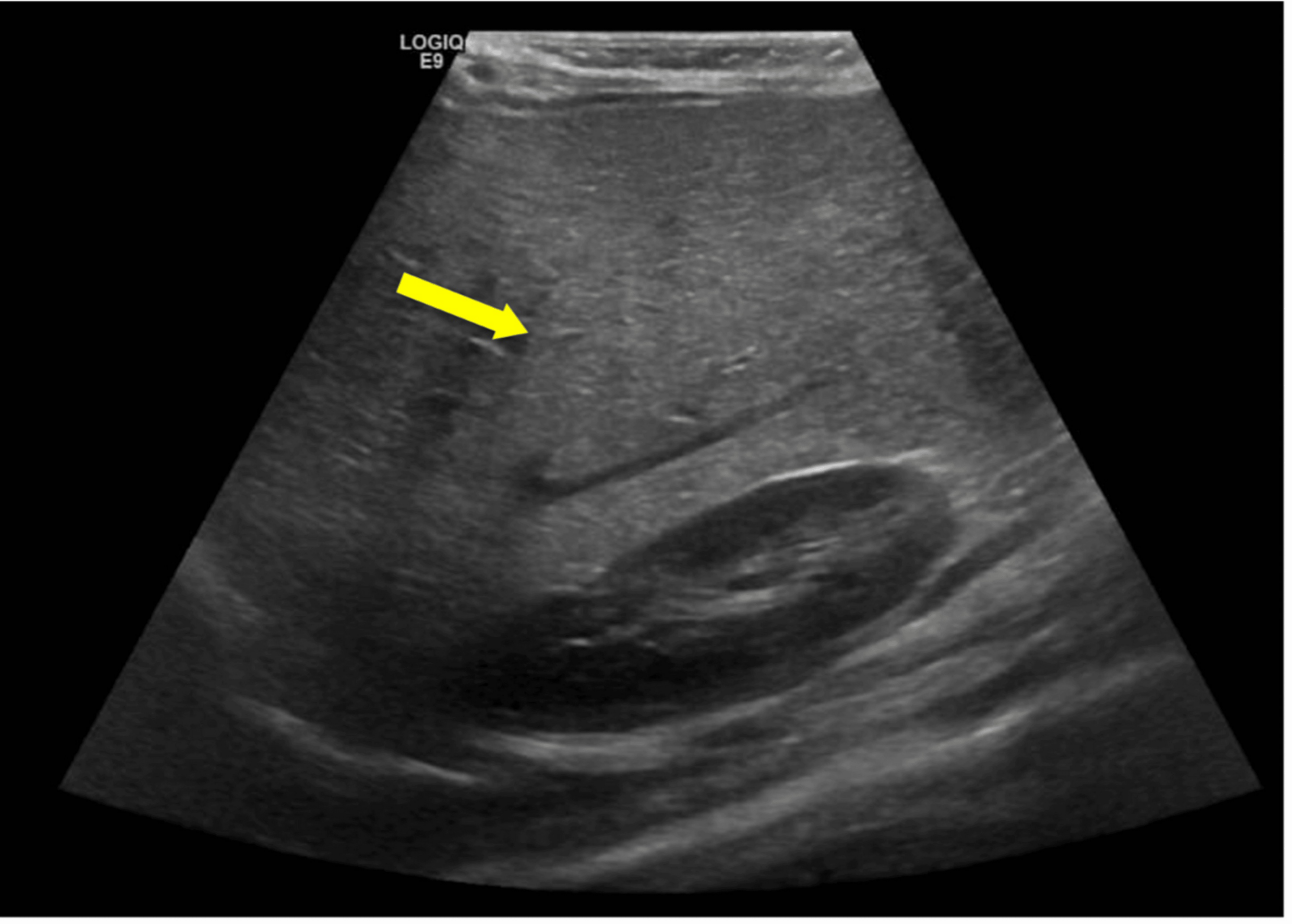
Abdominal ultrasonography image showing increased echogenicity of the liver compared to surrounding structures like the kidney, as indicated by the arrow, supporting the presence of a fatty liver.
